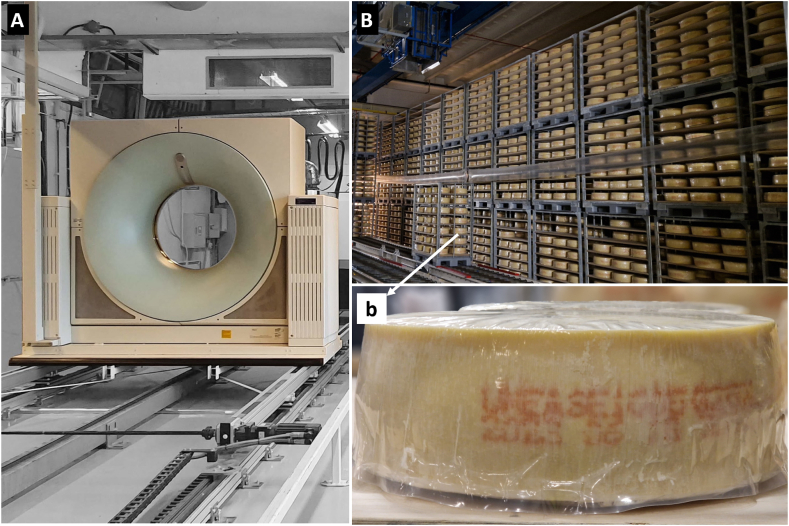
A: The Siemens Somatom Emotion Duo CT scanner (A) was used to scan the Grevé cheeses (b) in a non-destructive manner; the cheese was undisturbed, covered with wax and plastic cover during the scanning process. B: Grevé cheese ripening facility, where cheeses were stored for eye development and in between CT scanning.
